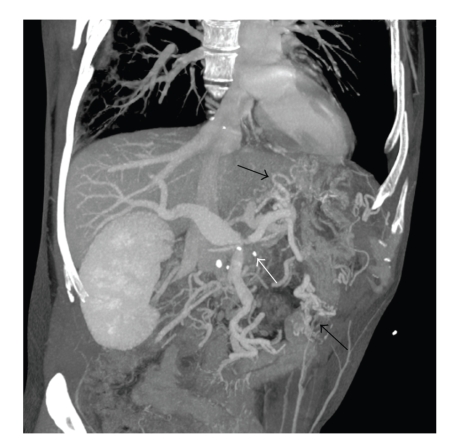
CT angiography coronal plane reconstruction showing the portal vein system with obstruction from the recurrent pancreatic tumor at the portal vein confluence (white arrow) and resulting gastric and small bowel varices (black arrows).
